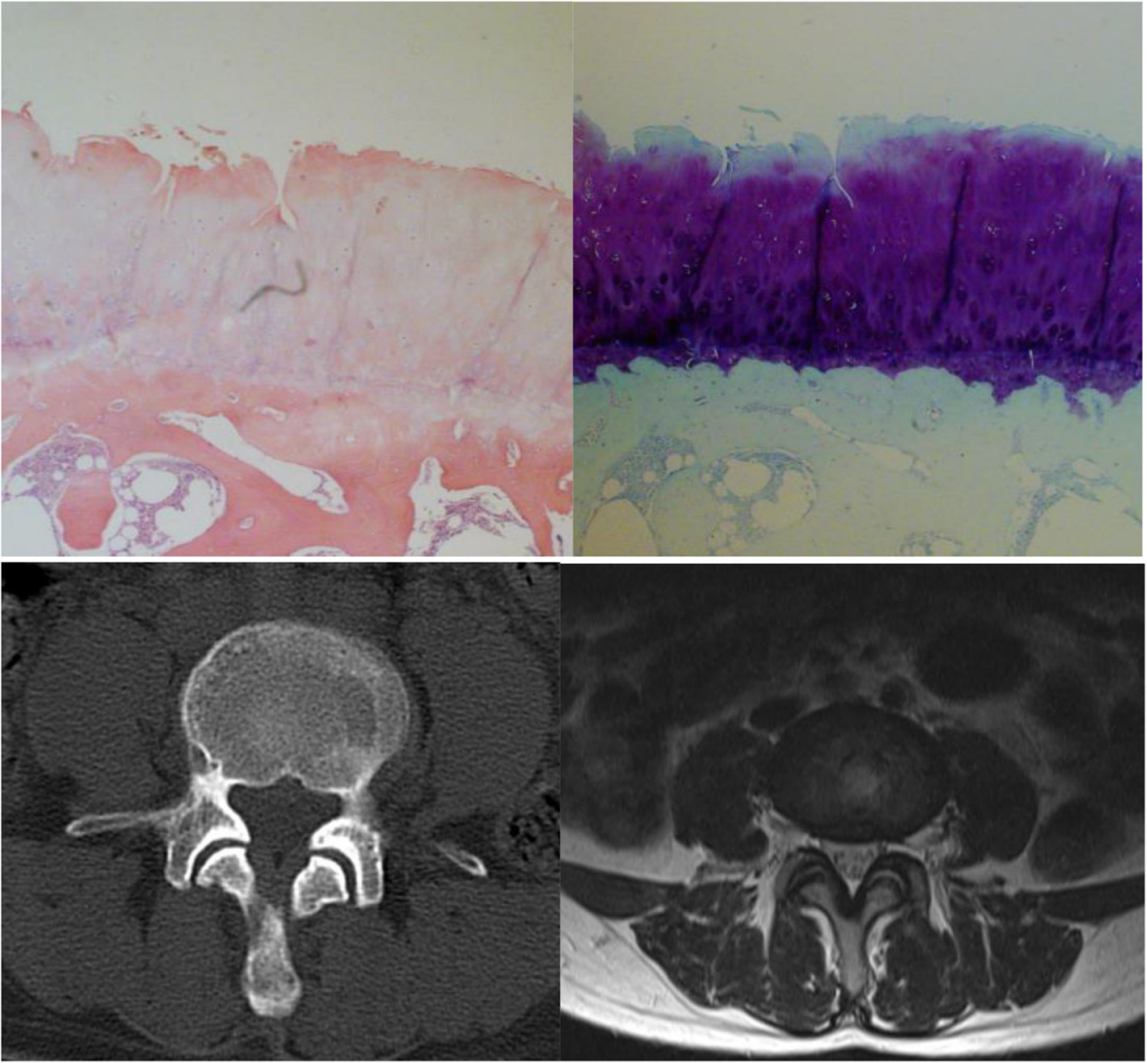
The pathologic grading of the left L4/5 facet joint was 3. While the CT and MR grading was 2, the CT combined MR grading was 3. Both the CT and the MR grading underestimated the degree of facet joints degeneration
